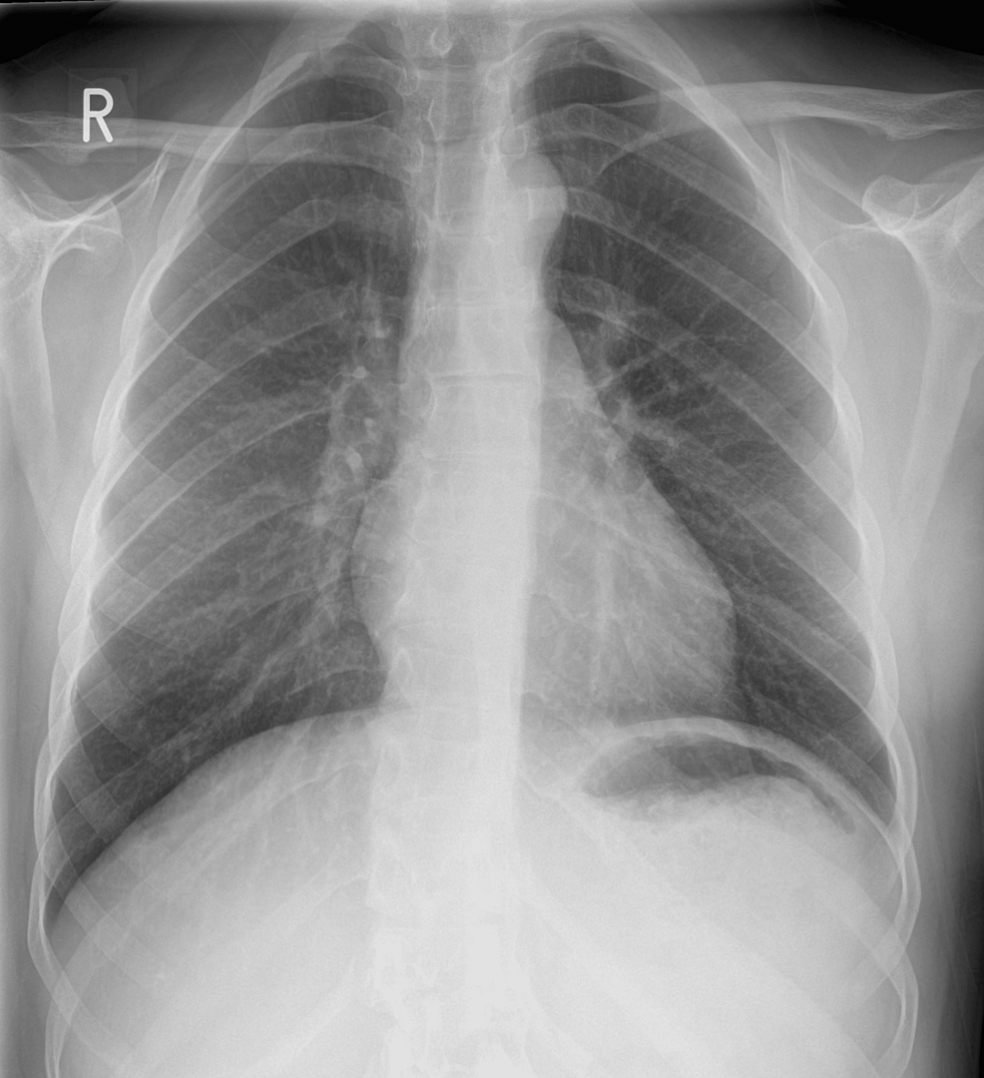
Chest X-ray before discharge showing clear lung fields (current admission).
